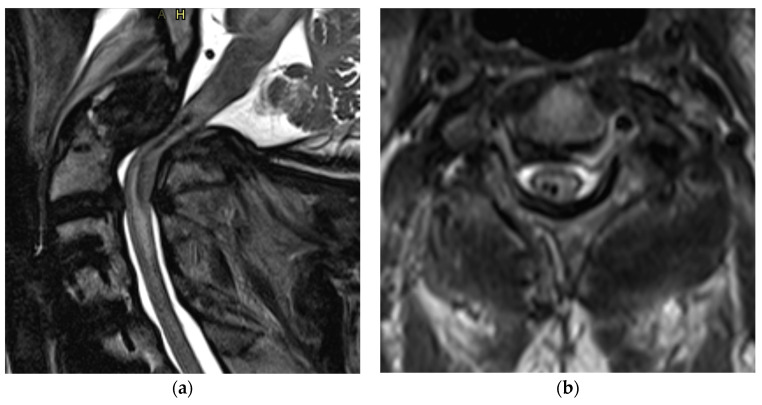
(a) Sagittal and (b) axial view of T2 MRI showing severe spinal cord injury with intramedullary hemorrhage.
